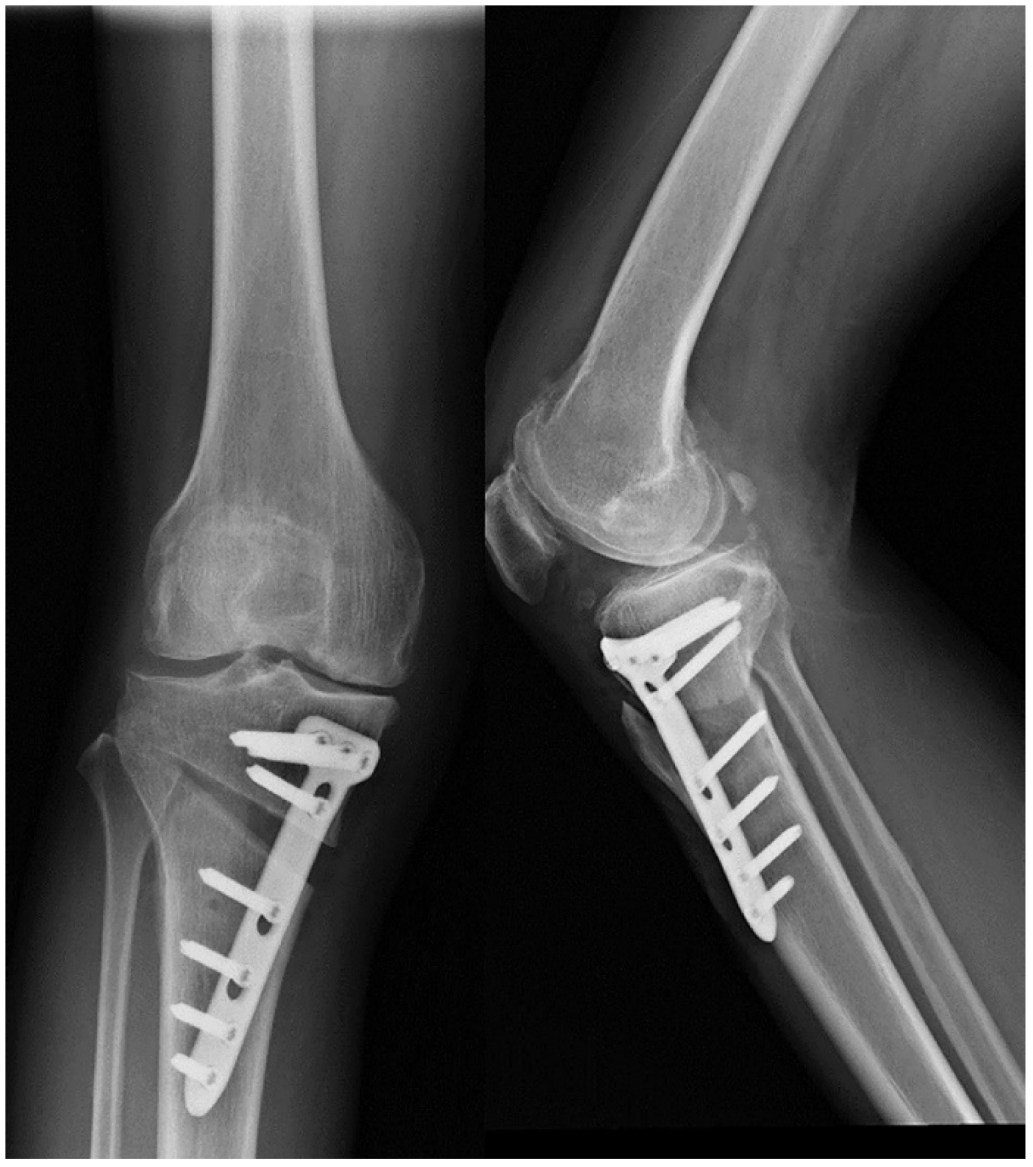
Postoperative X-ray of the knee joint in both anteroposterior and lateral positions after HTO.
